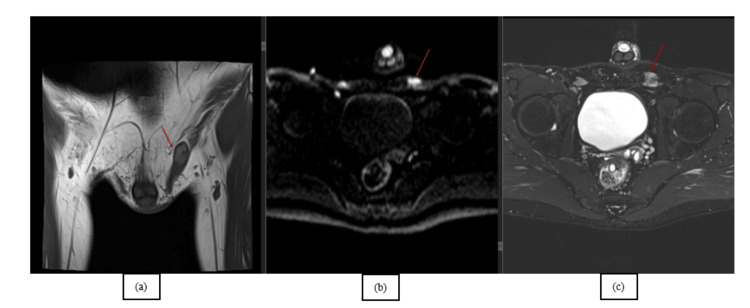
Coronal T1-weighted image (a), trace image (b), and T2FS (c) confirm the diagnosis of cryptorchidism with typical T1 appearance of the undescended testes and the spermatic cord; the high signal on the trace image is fully diagnostic of the testes. This figure is the original work of the authors. Patient consent for the use of the image was obtained, as mentioned in the patient consent form.
